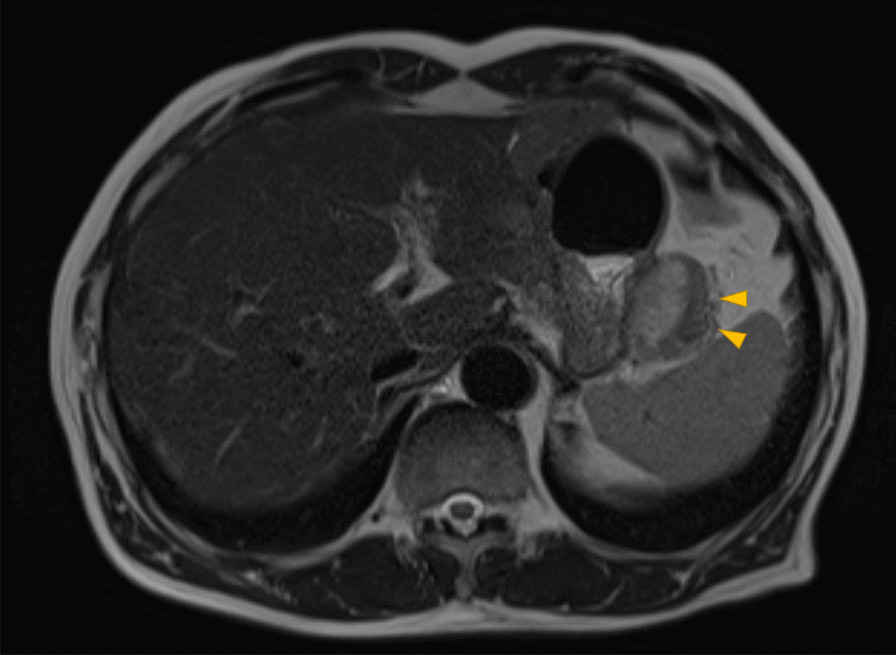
Magnetic resonance imaging. T2-weighted image showing a heterogeneous high signal. The arrows show the soft tissue mass
